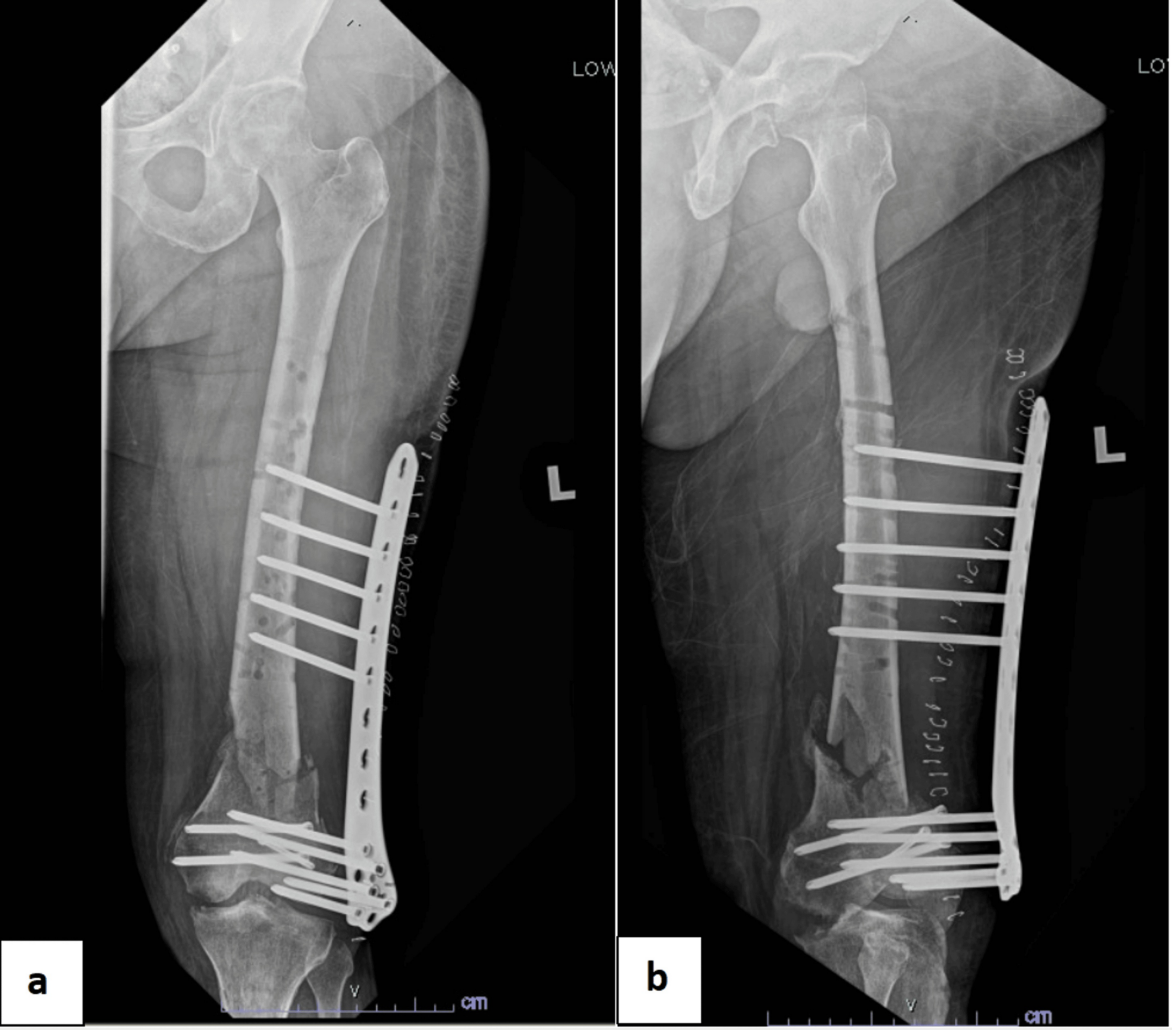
Radiograph of the left femur after supracutaneous plating of the left distal femur using a distal femur locking plate. (a) Anteroposterior view. (b) Lateral view.
